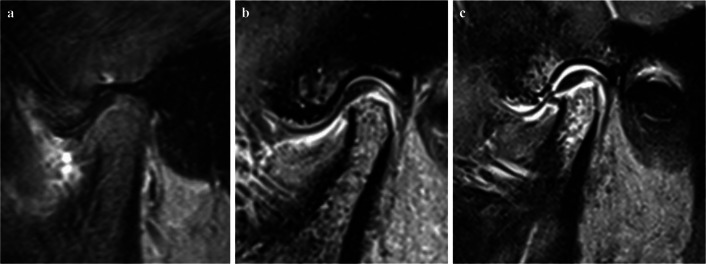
MRI assessment of TMJ involvement (sagittal oblique T1-weighted fat-saturated contrast-enhanced images), showing a) normal TMJ in a 12-year-old girl; b) mild to moderate inflammation and mild flattening of the condyle in a 16-year-old girl; and c) moderate to severe inflammation and a flattened condyle in a 15-year-old girl
